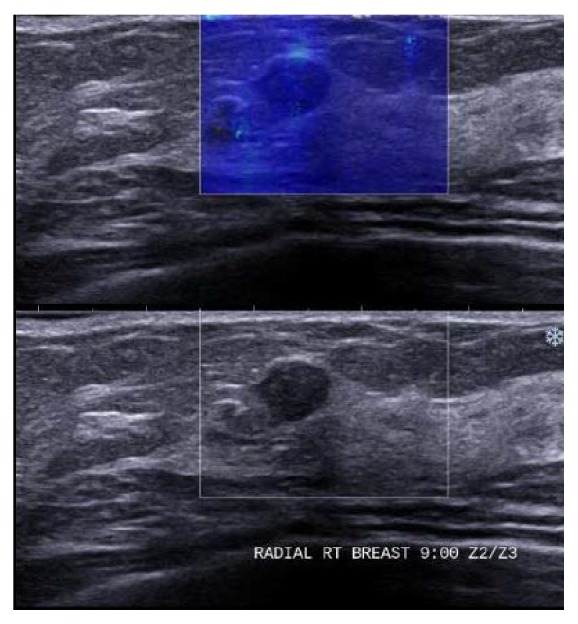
Target ultrasound corresponding to mammogram, there is partially marginated hypoechoic mass noted at 9 o'clock mid to posterior third of the right breast measuring 0.7 × 0.8 × 0.5 cm. This mass shows tubular extension from the lateral aspect and shows no internal vascularity. This mass appears relatively soft in elastography.
